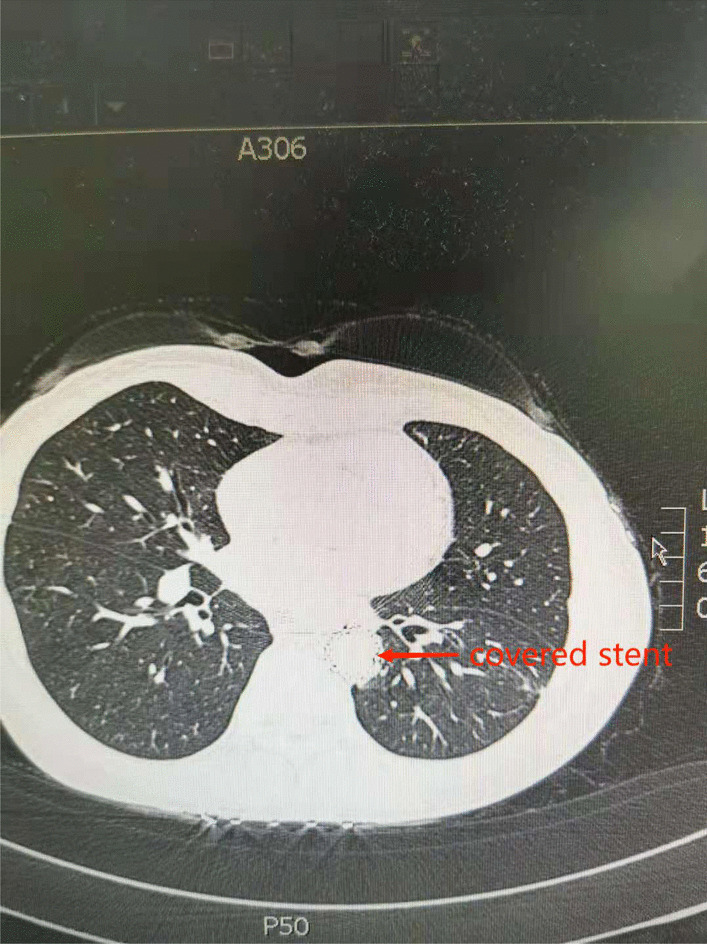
One-year after exploratory thoracotomy and TEVAR (Chest CT scan)
